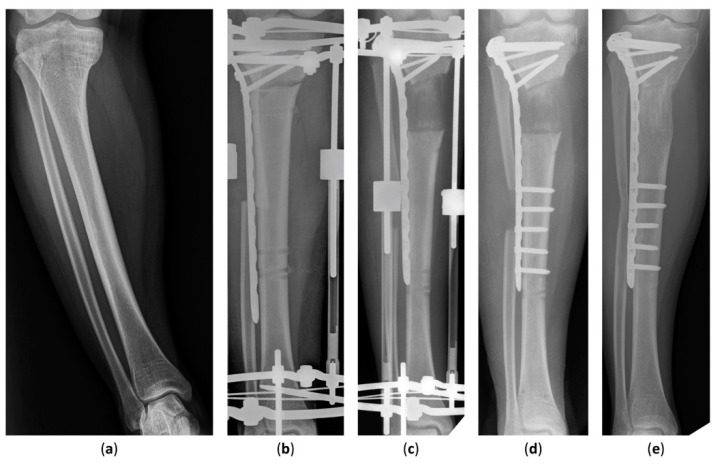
Anteroposterior radiographs of an 18-year-old girl who underwent fixator-assisted plating and acute angular correction for leg-length discrepancy. (a) A preoperative anteroposterior view of the tibia. (b) The procedure was conducted using an Ilizarov apparatus and a submuscular plate. (c) Satisfactory tibial lengthening and alignment were achieved. (d) The distal part of the submuscular plate was fixed at removal of the external fixator. (e) The distracted callus healing with good consolidation was seen in the latest radiograph 40 months after the operation.
